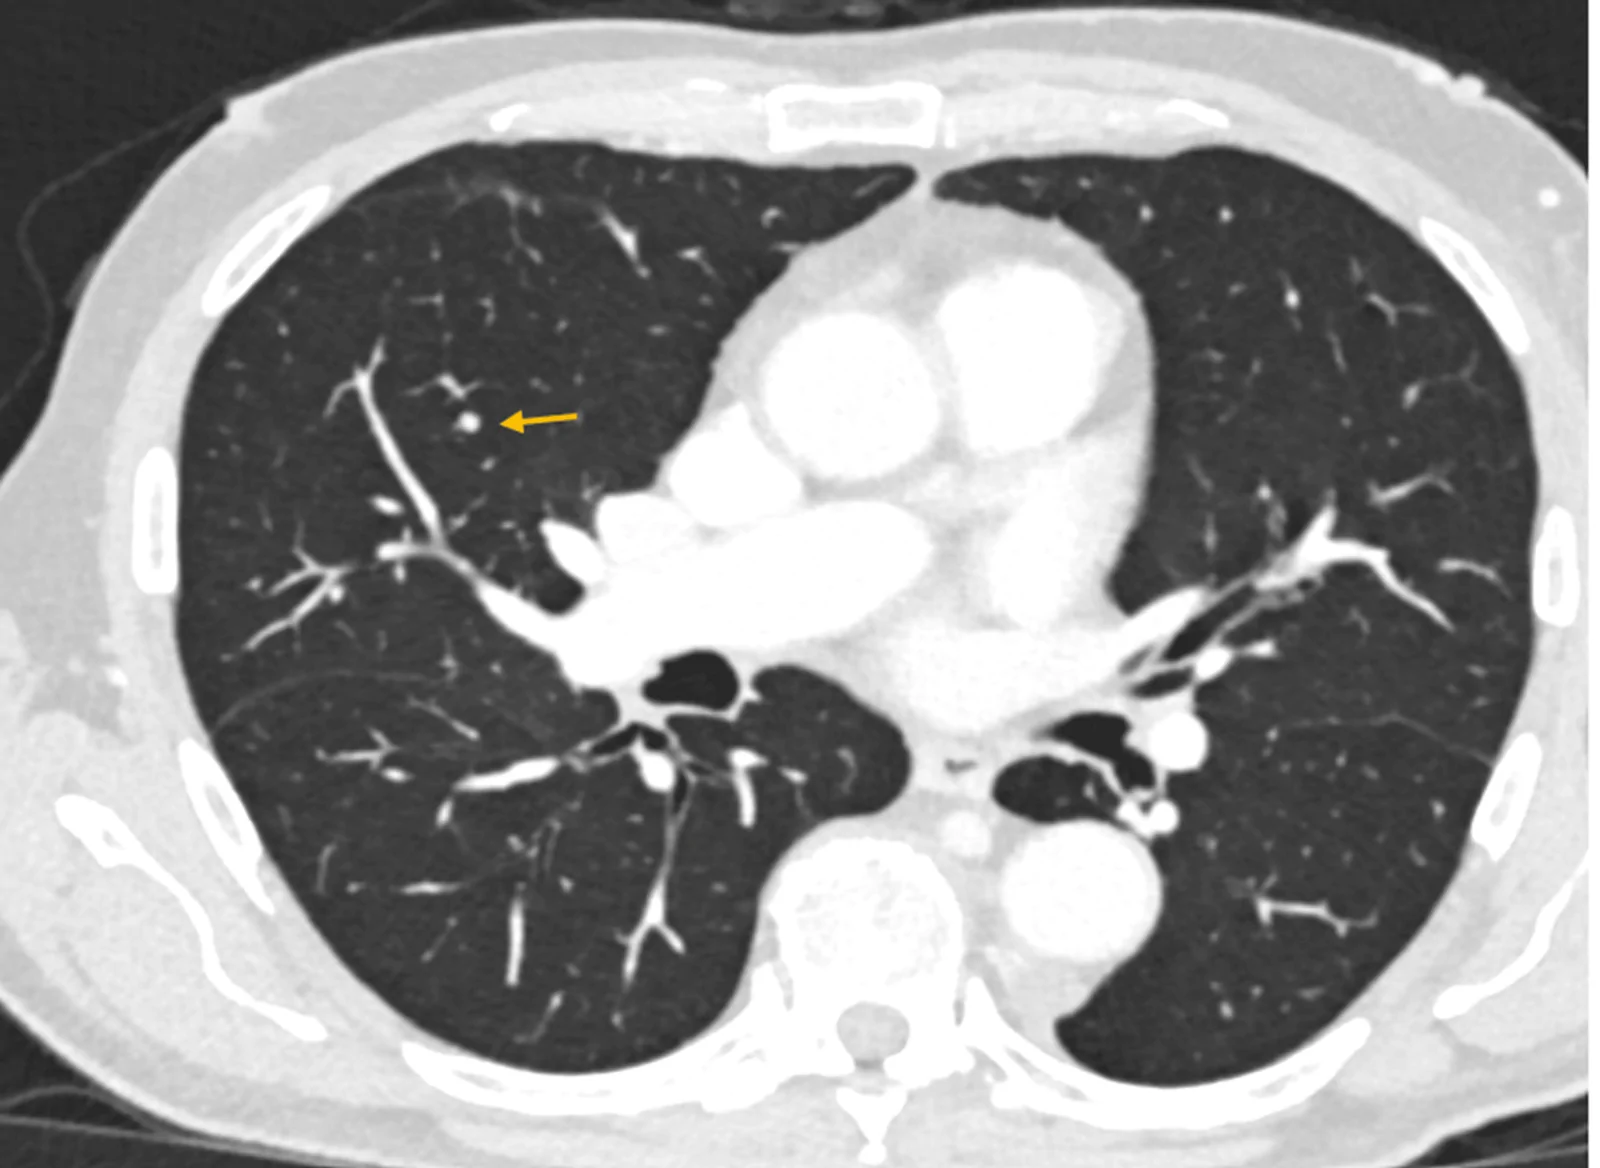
Contrast-enhanced axial CT image showing right lung metastases (yellow arrow).
